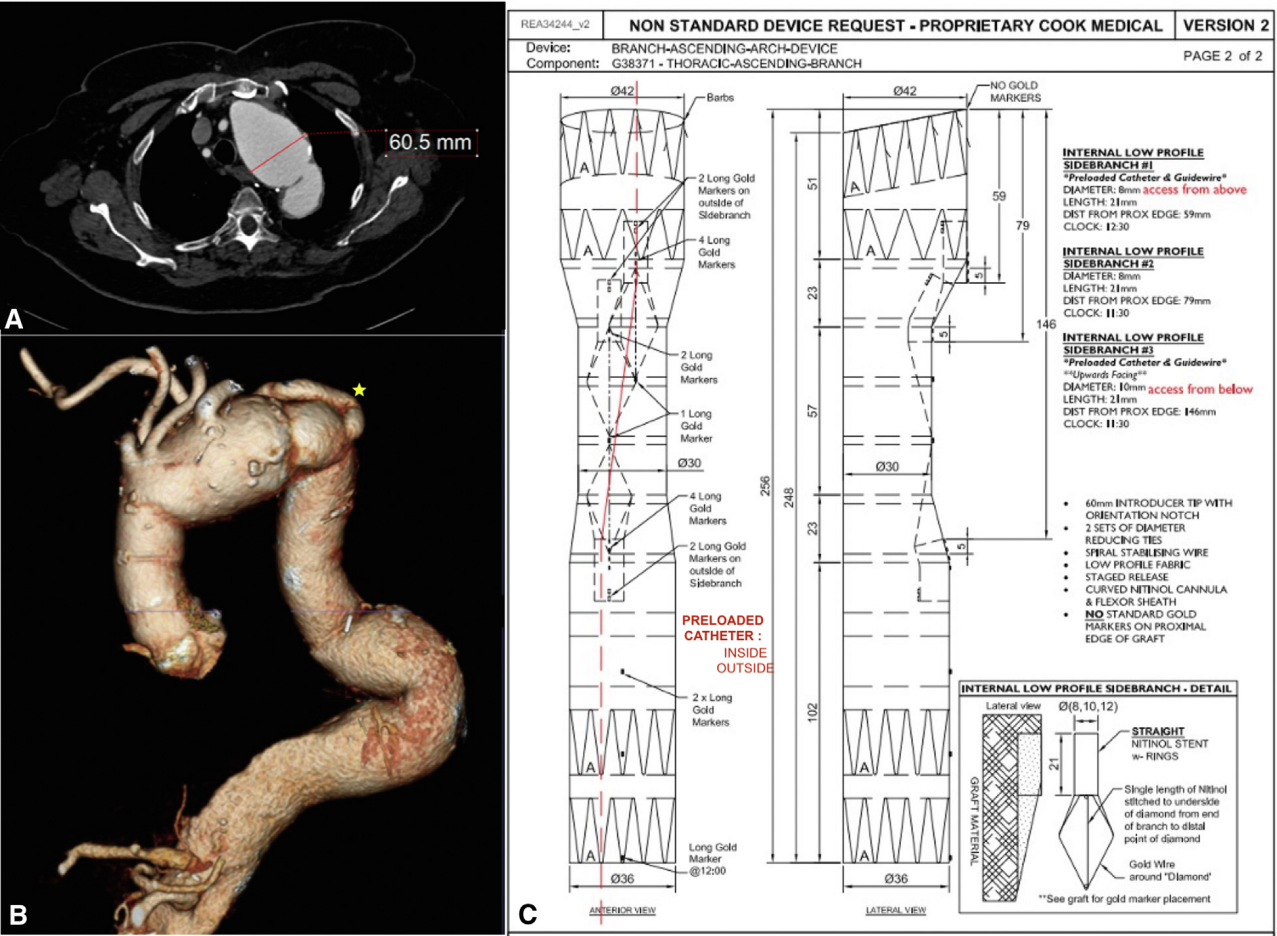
A, Three-dimensional computed tomography angiography demonstrated a 61-mm aortic arch aneurysm. B, Retroesophageal aberrant right subclavian artery (*yellow star*) was reimplanted on the descending thoracic aortic graft, with a posterior and kinked takeoff. C, Graft plan.
